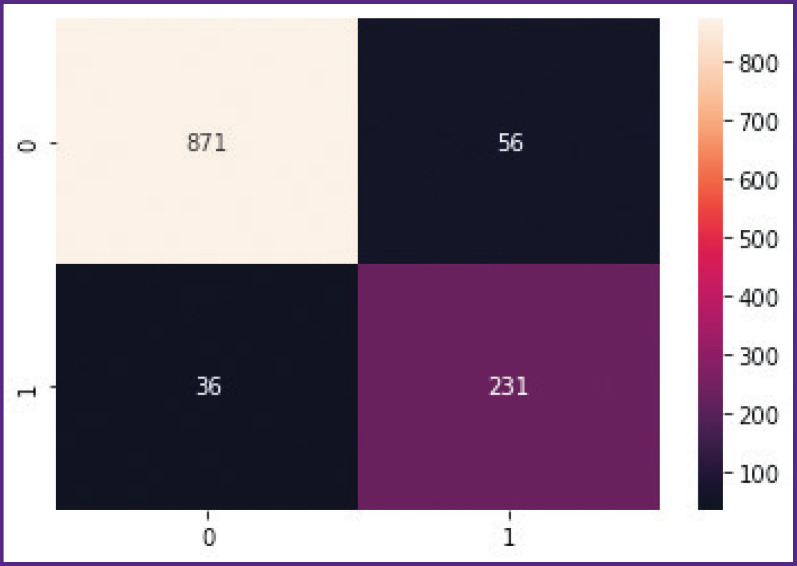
Error matrix: vertically — true estimate of CT investigation: 0 — investigation without signs of intracerebral hemorrhages (true-negative result); 1 — investigation with signs of hemorrhages (true-positive result); horizontally — estimates using the developed algorithm: 0 — presence of pathology was found erroneously (false-positive result); 1 — absence of pathology was indicated erroneously (false-negative result)
